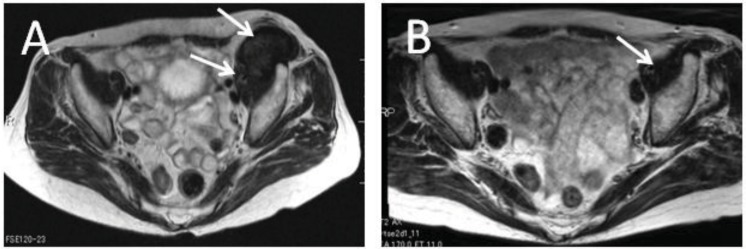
Seventy-one years old, female with desmoid (arrows) in left iliopsoas muscle. (A) T2-weighed axial image of MRI before meloxicam treatment. (B) MRI of the same patient after 54 months’ treatment with meloxicam.
